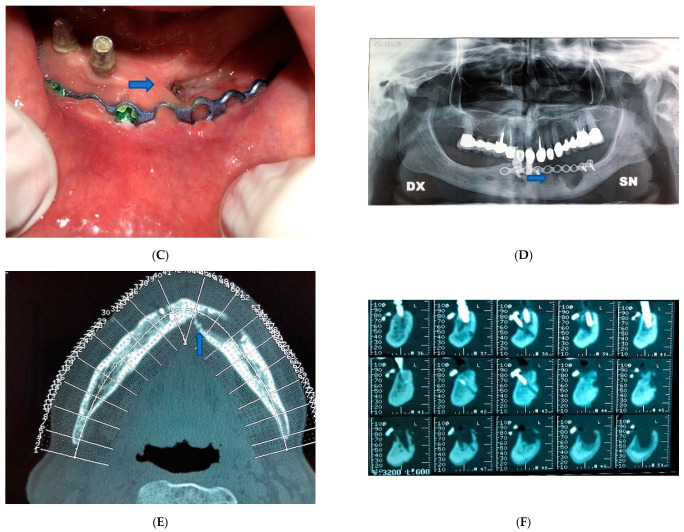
Patient 2. (A) Pre-operative panoramic X-ray examination showed no fractures, but there was a subversion of the normal organization of the bone. (B) Pre-operative X-Ray image showed lithic area in the lower branch. (C) Epi-mucosal fixation performed using SMART Lock screws and plates without elevating the mucoperiosteal flap. (D) Post-operative panoramic X-ray image of the dental branch revealed lithic area. (E) Post-operative CT scan Image. (F) Post-operative X-ray image.
